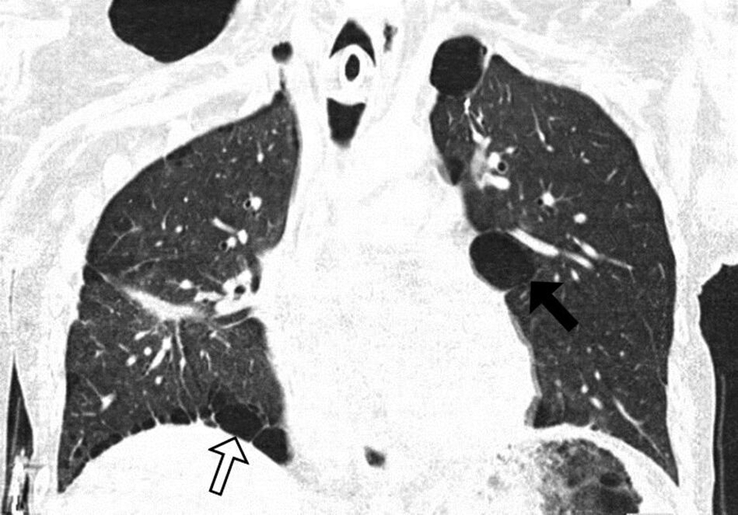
Chest computed tomography scan; coronal view: multiple irregular shape, variable size cystic disease with thin well-defined walls, more pronounced in the right lower lobe, subpleural (white arrow) and peri-mediastinal in location.
